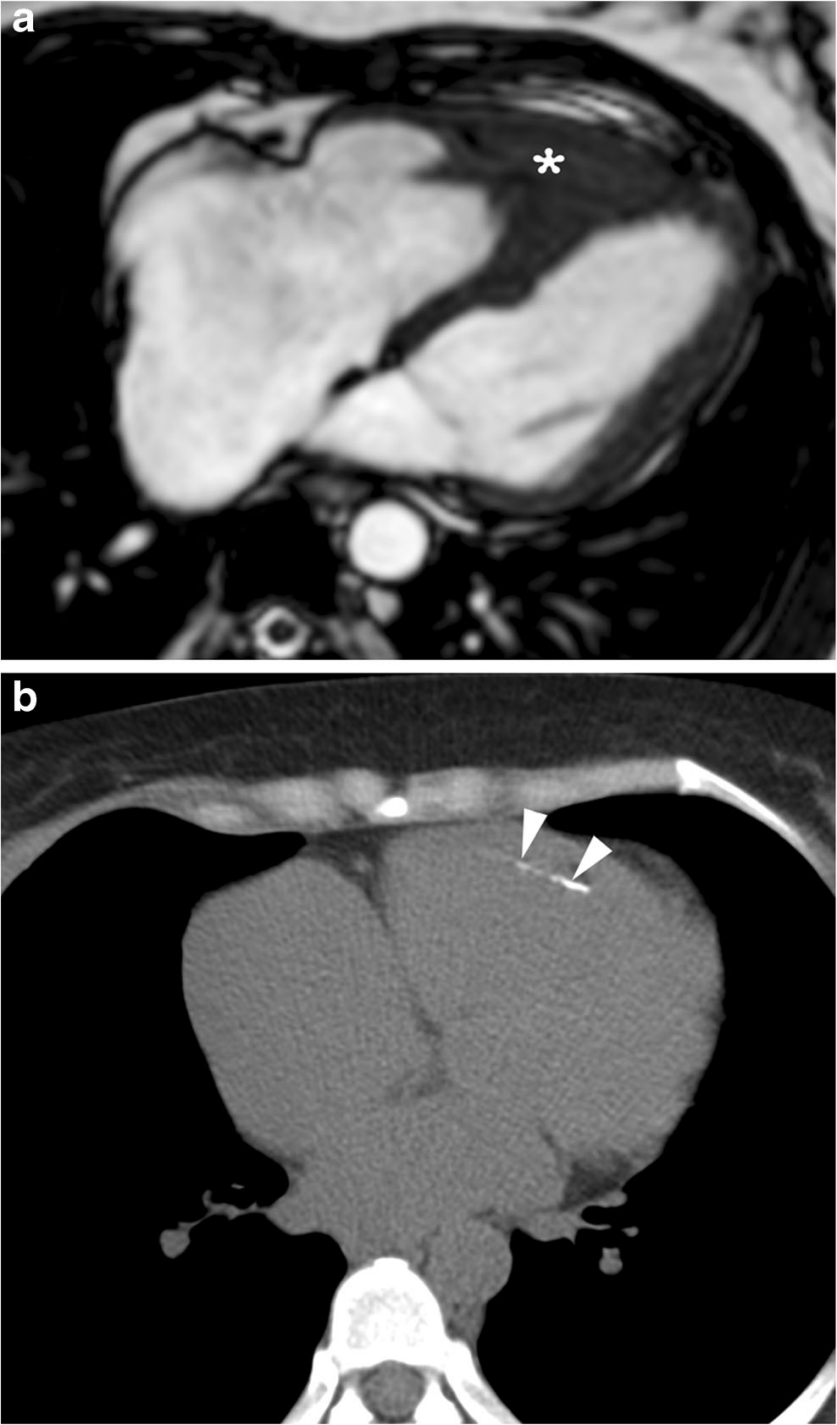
A 39-year-old woman with endomyocardial fibrosis. a Four-chamber view cine SSFP MR image at end-diastole shows right atrial dilatation and myocardial thickening (*asterisk*) with obliteration of the right ventricular apical chamber. b Non-enhanced thoracic CT scan showing endocardial calcification (*arrowheads*)
